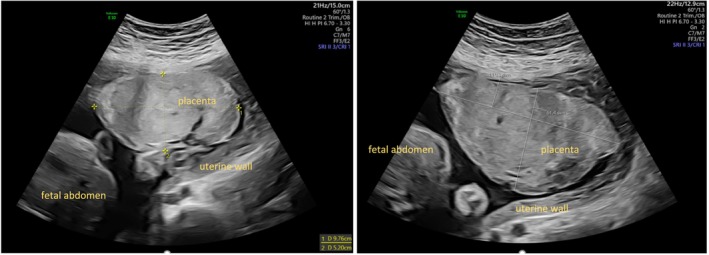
Ultrasound placenta case 1 (29 + 3 and 31 + 3 weeks of gestation). The placenta has a small adhesive surface and is thickened.
